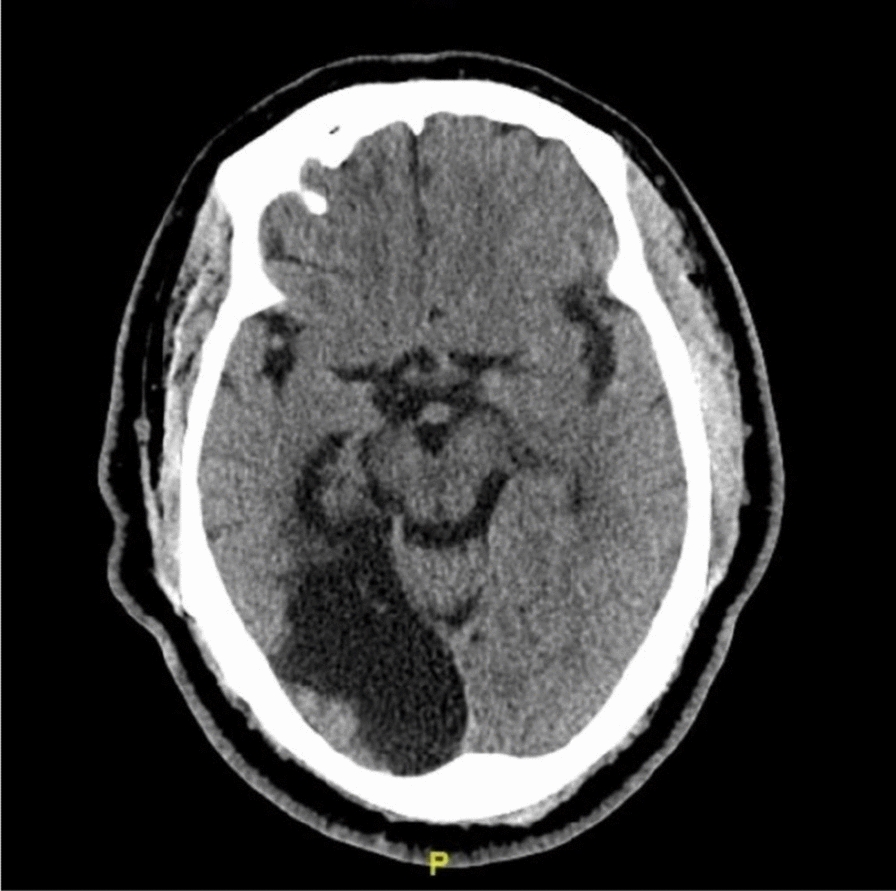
CT scan of old posterior cerebral artery (PCA) territorial infarct involving occipital & temporal lobes.[75] Copyright © 2019, Gheewala et al.
